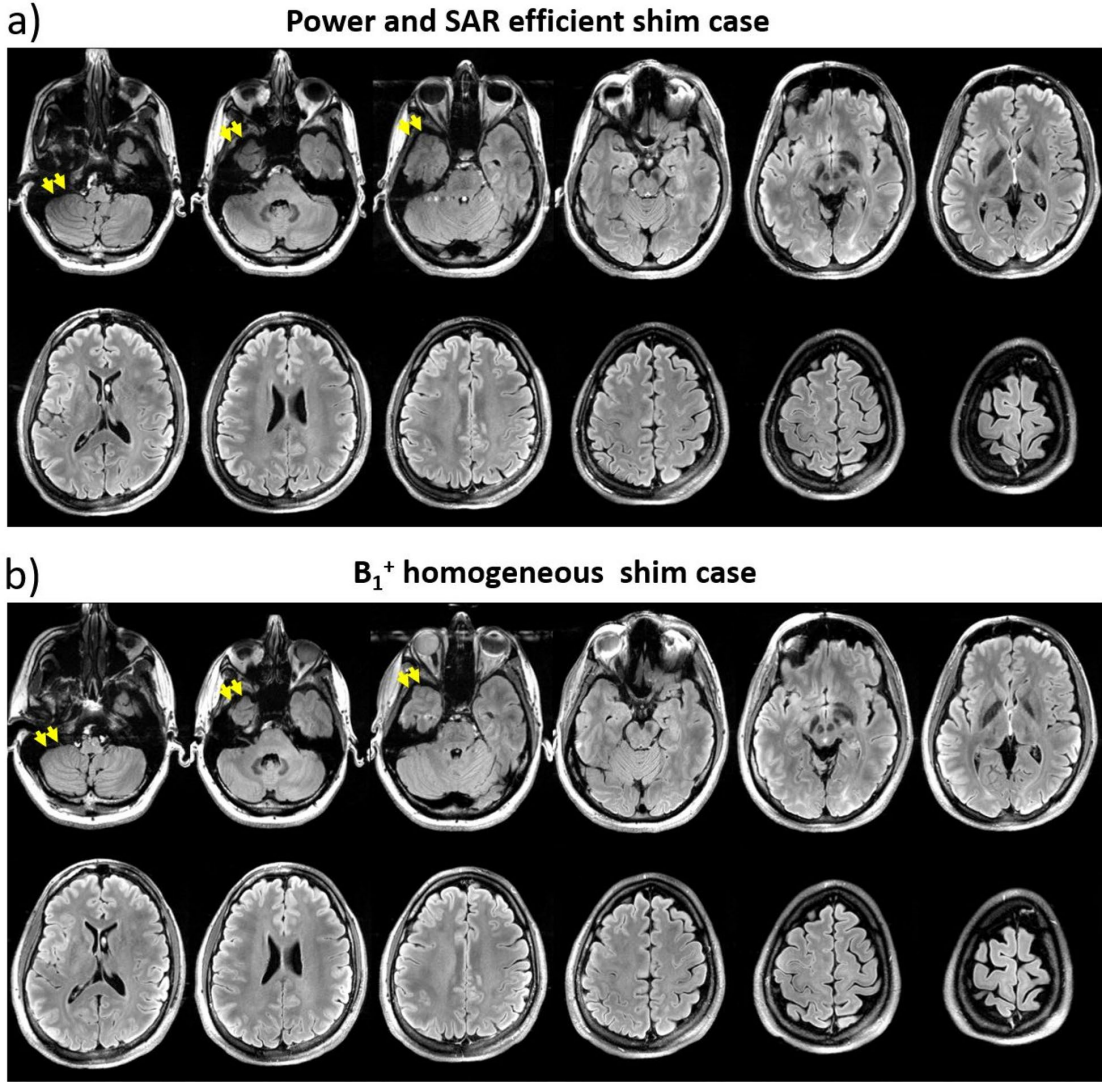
Axial slices of the FLAIR images acquired at resolution of 0.7 × 0.7 × 2 mm^3^. The images show the differences between the power and SAR efficient (a) and B_1_^+^ homogeneous (b) shim cases. The yellow arrows point to the regions where the dropout was mitigated by the B_1_^+^ homogeneous case. The parameters of the acquisition were: TE/TI/TR = 103/2900/13,500 ms, acceleration factor 2, BW = 230 Hz/Px, transversal acquisition of 64 slices, field of view 224 × 176.4 mm^2^ in axial plane, and acquisition time = 7:36 min.
